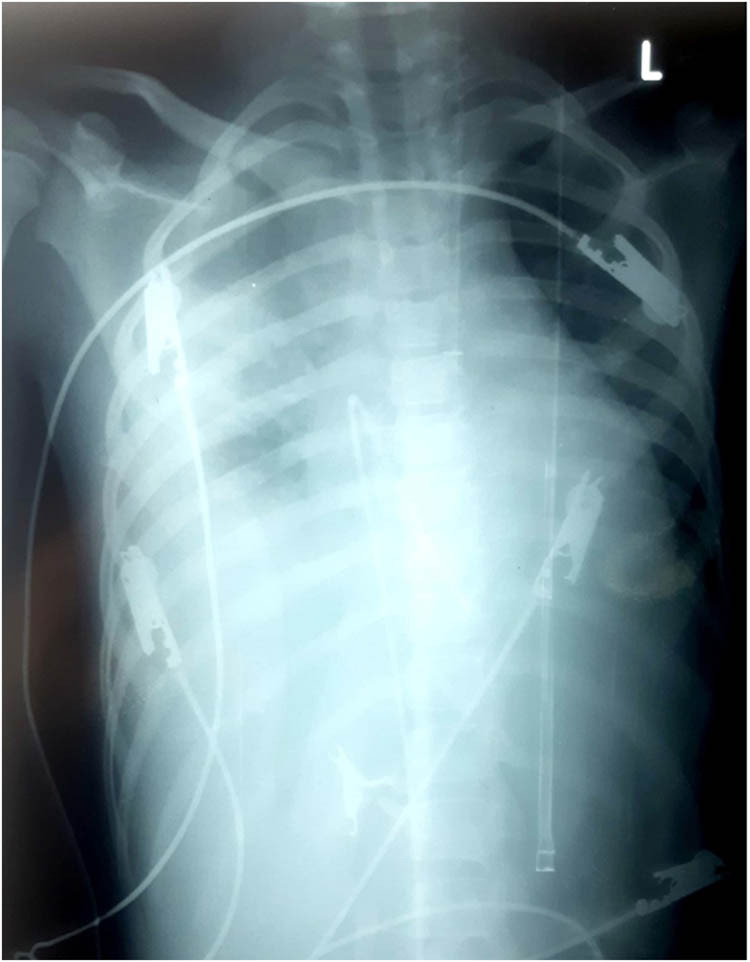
Chest X-ray showing pleural effusion and cardiomegaly.
